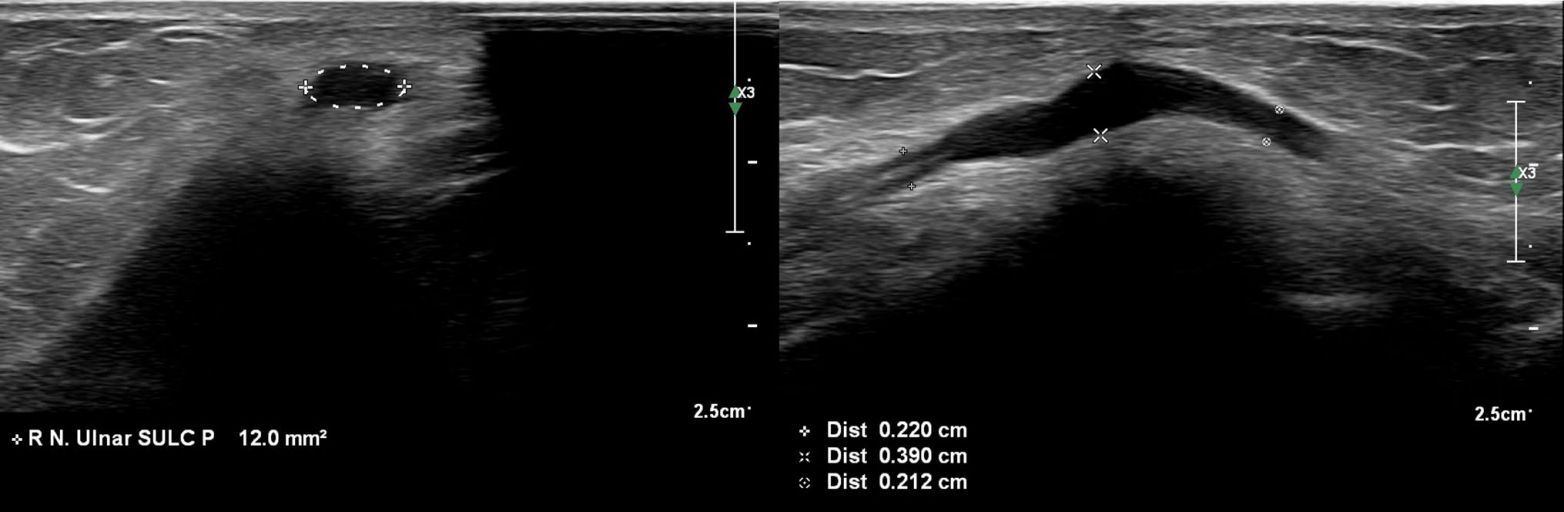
Ultrasound image of the ulnar nerve at the cubital tunnel. Left: a cross-sectional view of the ulnar nerve at the cubital tunnel. Right: a longitudinal view of the ulnar nerve at the cubital tunnel where thickening of the nerve is shown
